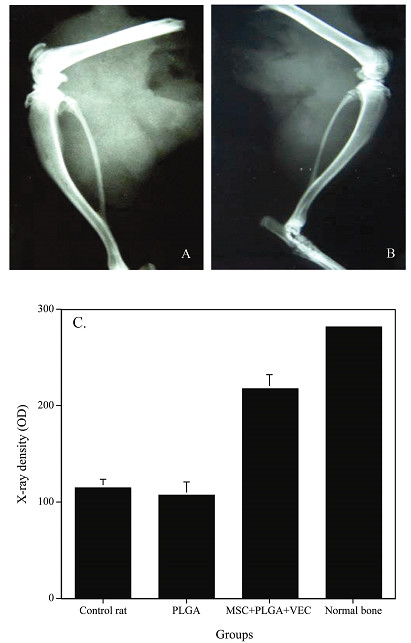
Soft X-ray images from right and left thigh and X-ray density analysis using NIH image software. A. Right thigh implanted with the PLGA seeded with the MSCs and VECs. B. Left thigh implanted with the PLGA seeded with the MSCs. C. X-ray density analysis. The data shown are the means ± SD from six experiments.
